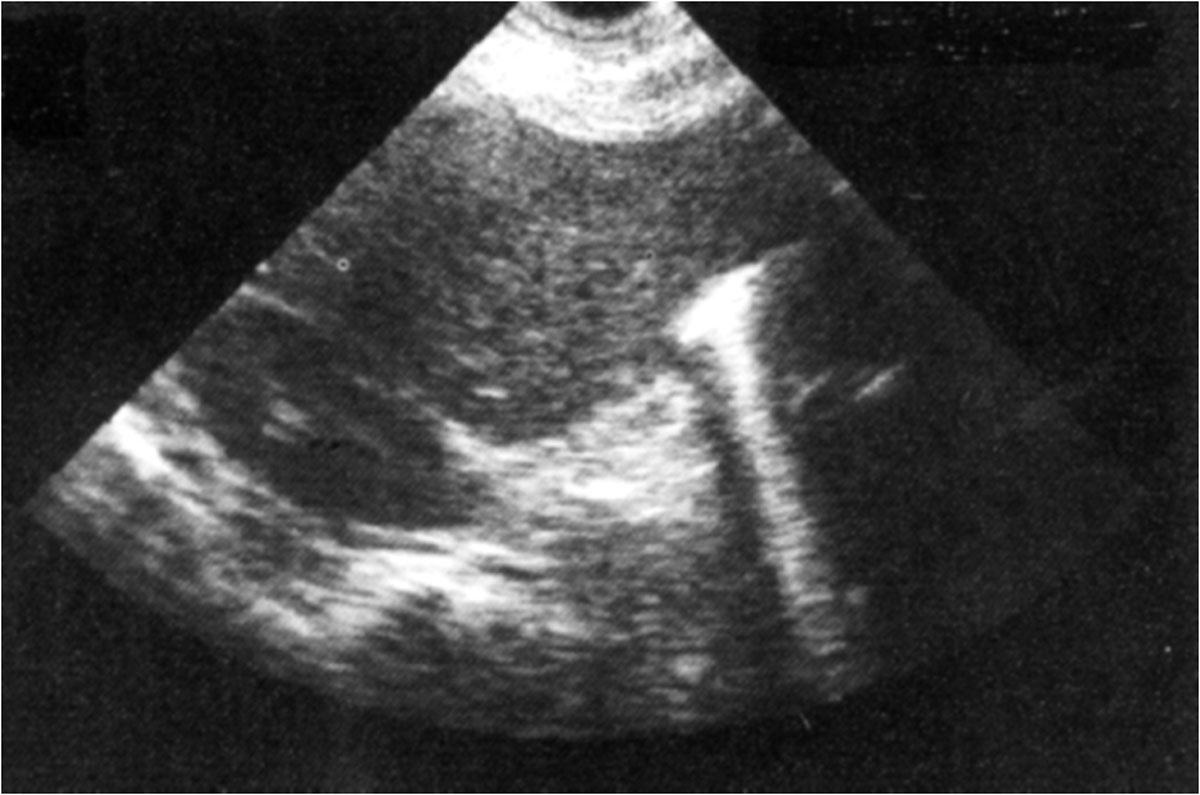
The sonographic appearance of free intraperitoneal air results form scattering of the ultrasound waves at the interface of soft tissue and air which is accompanied by reverberation of the waves between the transducer and the air
